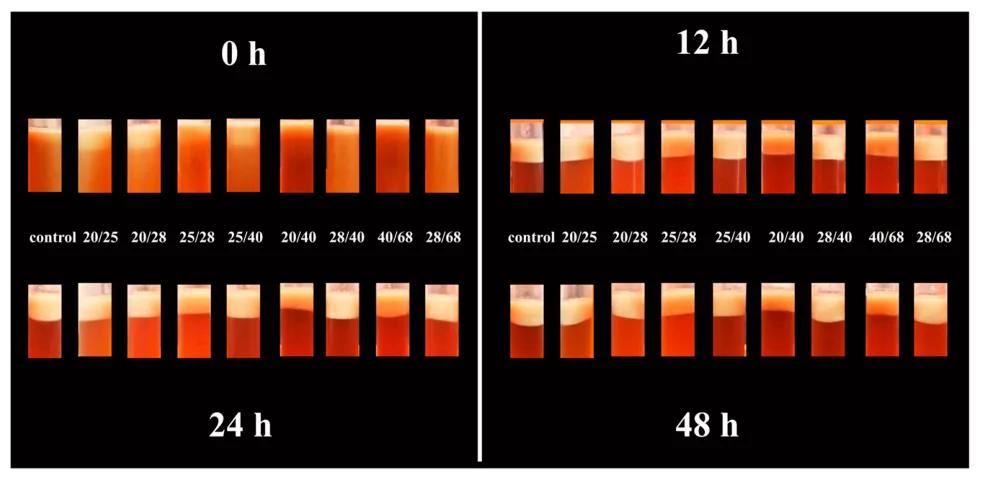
Representative photographs showing the storage stability of beef MP emulsions prepared after dual-frequency ultrasound-assisted glycation during storage for 0, 12, 24, and 48 h.
